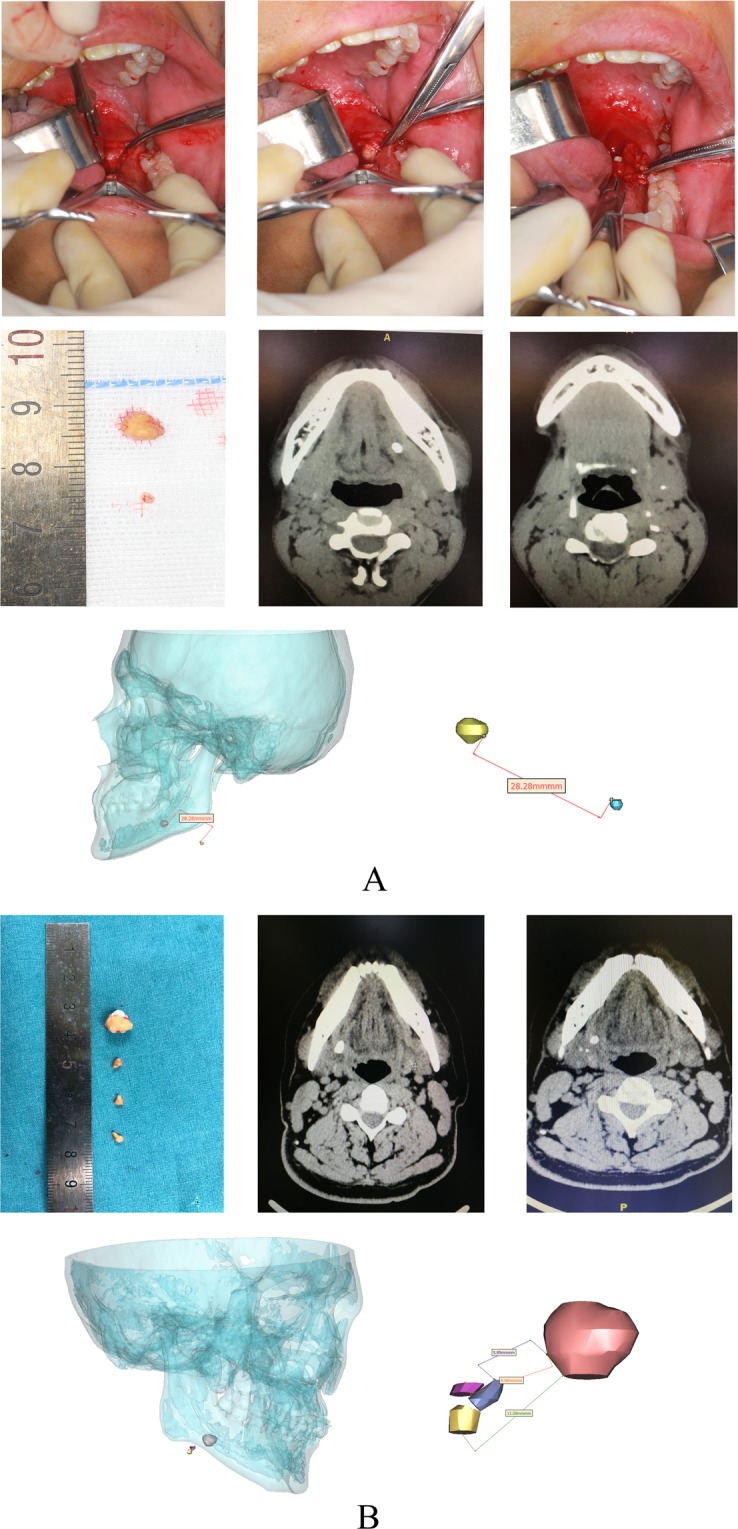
Intraoperative photographs and CT three-dimensional reconstructions of Case 1 and Case 2.
